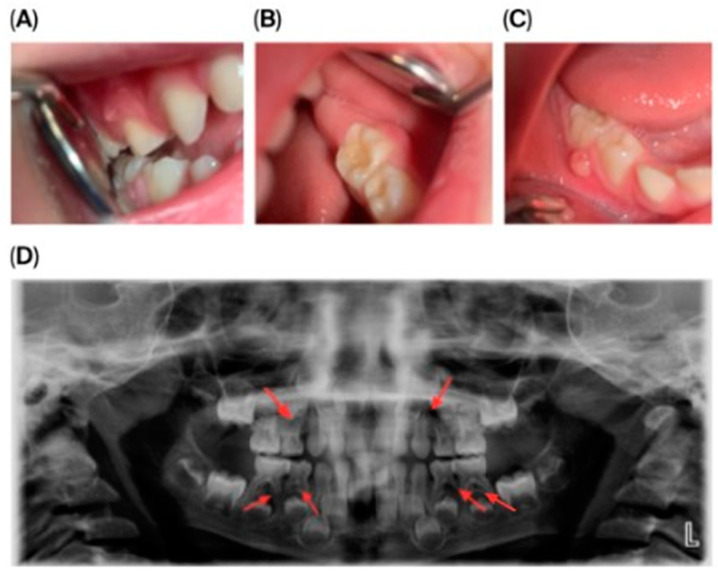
Intraoral and extraoral photographs and panoramic imaging of the patient showing multiple fistulas and dental pathology: (A) Buccal fistula in the upper right quadrant. (B) Buccal fistula in the lower left quadrant. (C) Buccal fistula in the lower right quadrant. (D) Panoramic radiograph (2024) showing extensive periapical radiolucency and root resorption in multiple primary molars (54, 64, 74, 75, 84, 85).
